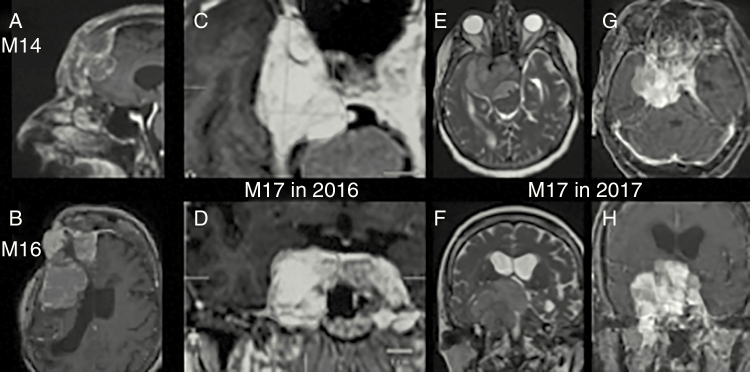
Patients with positive liquid biopsy. (A and B) Patients M14 (A) and M16 (B): 3D T1-weighted with gadolinium enhancement MRI. In both cases, the *NF2* mutation was detected in cfDNA. A subcutaneous extension was observed on MRI. (C–H) Patient M17: C and D display axial and coronal 3D T1-weighted with gadolinium enhancement MRI in 2016; E and F display axial and coronal T2-weighted MRI in 2017; G and H represent axial and coronal 3D T1-weighted with gadolinium enhancement MRI in 2017. We observed a major progression comparing tumor volume in 2016 and 2017. *NF2* mutation at a high allelic frequency (28.7%) was detected in the CSF.
